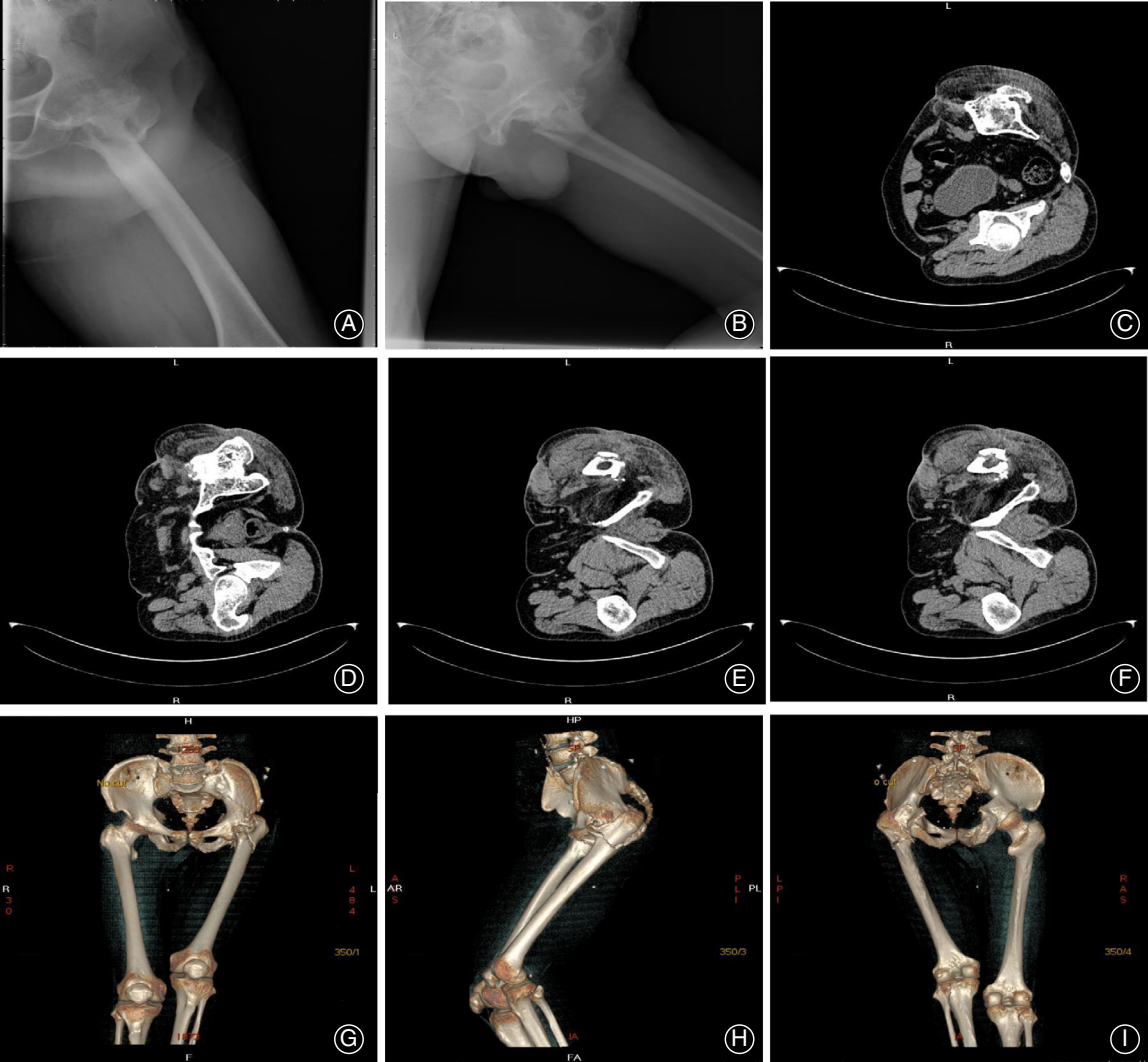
Preoperative imaging examination of the hip for the patient: (A) Anterior radiograph of the left hip joint; (B) lateral radiograph of the left hip joint; (C, D) preoperative CT images showing left hip fusion; (E, F) preoperative CT showing an intertrochanteric fracture of the left femur; and (G‐I) CT three‐dimensional reconstruction of the pelvis and femur.
